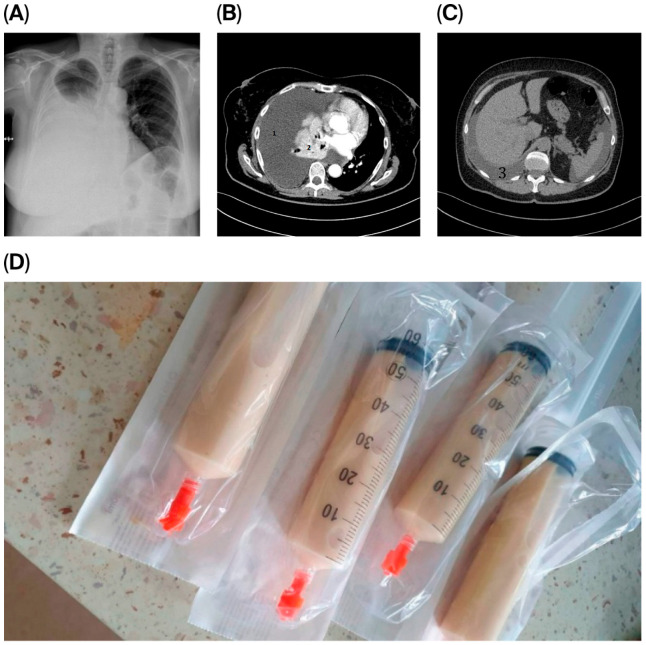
(A) A chest X-ray. A large amount of fluid in the right pleural cavity. (B) CT of the chest. Pleural effusion in the right pleural cavity (1) and atelectasis of the lower lobe of the right lung (2). (C) CT of the abdomen. Fluid in the peritoneal cavity next to the liver and spleen (3). (D) Milky fluid obtained during thoracocentesis. CT: Computed Tomography.
